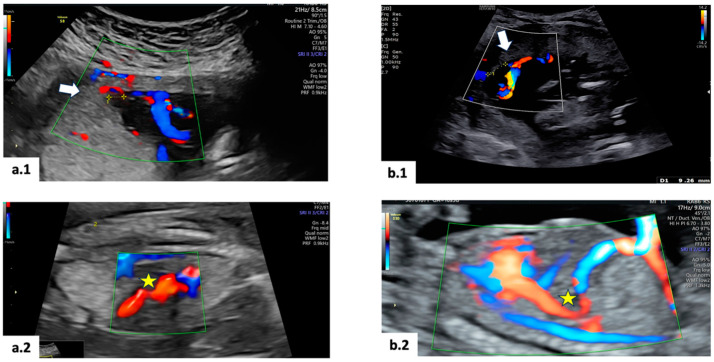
Main ultrasound characteristics: (a.1) velamentous insertion highlighted by ➭ and (a.2) ADV and UV drainage in the IVC highlighted by ★, in the first case; (b.1) marginal insertion highlighted by ➭ and (b.2) ADV and UV drainage in the IVC highlighted by ★, in the second case.
